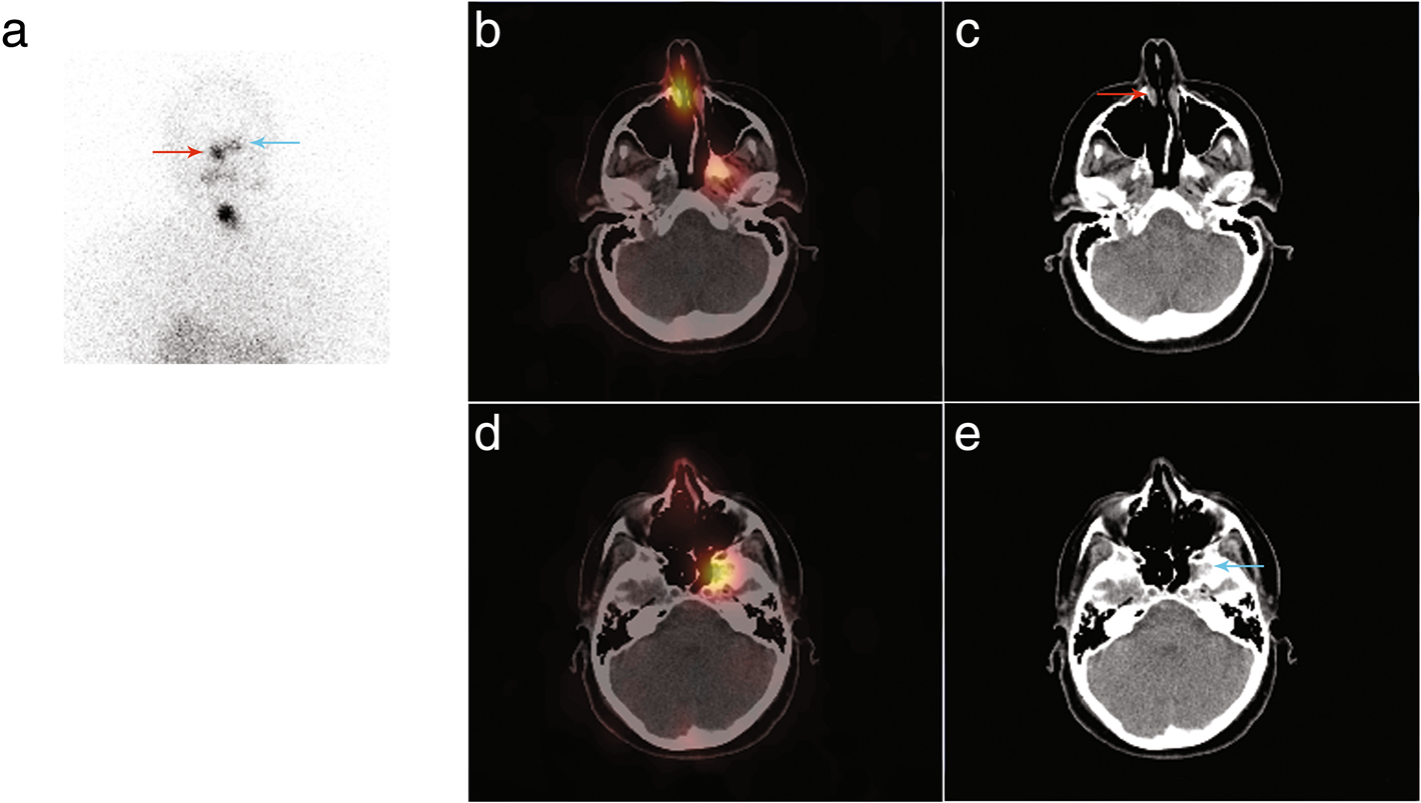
Planar acquisition (anterior view, Panel a), fused (Panel d) and CT (Panel e) images of SPECT/CT acquisition displaying RAI uptake in the left sphenoid sinus with mucosal thickening on CT scan slices (*blue arrow*). RAI uptake is also present in the nose (Panels a, b and c, *red arrow*)
